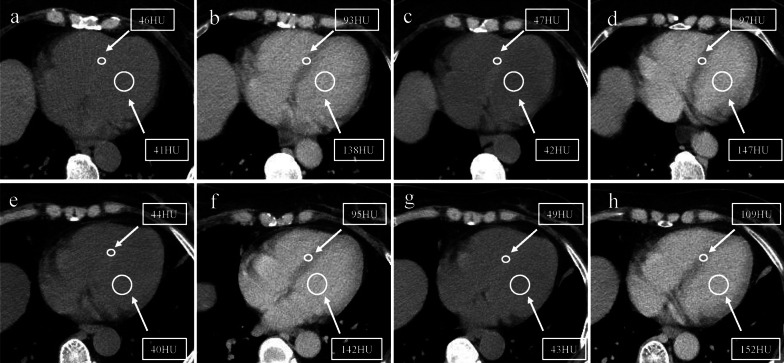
A 48-year-old BC patient without CTRCD (a, b, c, d) and a 52-year-old BC patient with CTRCD (e, f, g, h). The baseline ECV value before anthracycline chemotherapy in patient without CTRCD was 29.56% derived from unenhanced (a) and enhanced CT images (b). The ECV value after completing anthracycline chemotherapy was 30.00% derived from unenhanced (c) and enhanced CT images (d). The baseline ECV value before anthracycline chemotherapy in patient with CTRCD was 29.50% derived from unenhanced (e) and enhanced CT images (f). The ECV value after completing anthracycline chemotherapy was 34.68% derived from unenhanced (g) and enhanced CT images (h). *BC*, breast cancer; *CTRCD*, cancer therapy-related cardiac dysfunction; *ECV*, myocardial extracellular volume; *CT*, computed tomography
